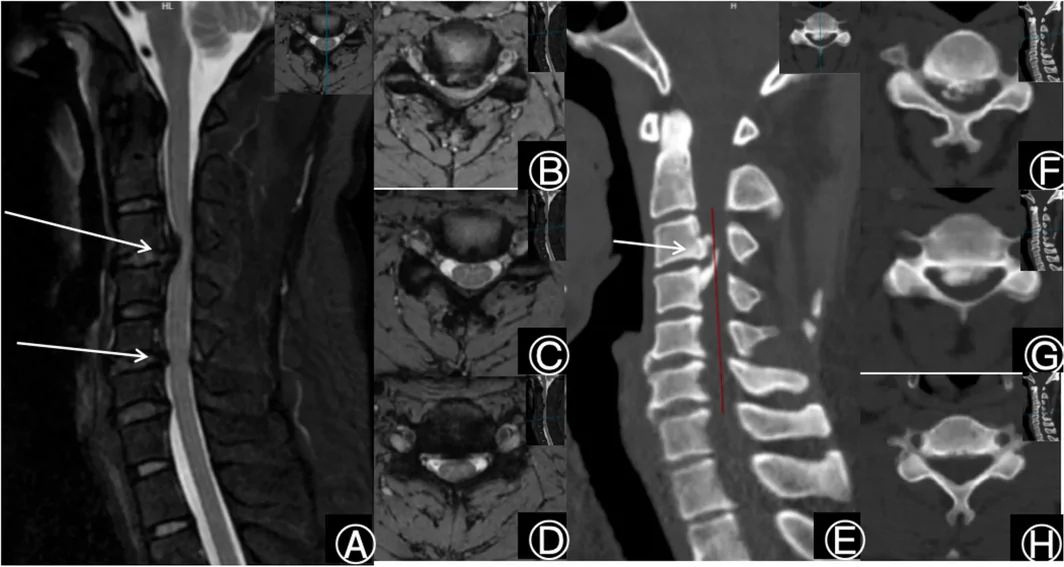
(A) Sagittal cervical MRI T2 view demonstrates multi‐level stenosis from C3 to C7. The axial view revealed the spinal canal being occupied by the ossific ligaments, along with lateral recess stenosis (B–D arrow). Cervical spine CT scan revealed OPLL posterior to C3 and C4 vertebrae. (E) Sagittal reconstruction CT. (F–H) Axial CT cuts of C3_C5. K‐line is negative (E, red line). OPLL posterior to the C3 and C4 vertebrae, with a canal occupying ratio of approximately 60% (F, G).
